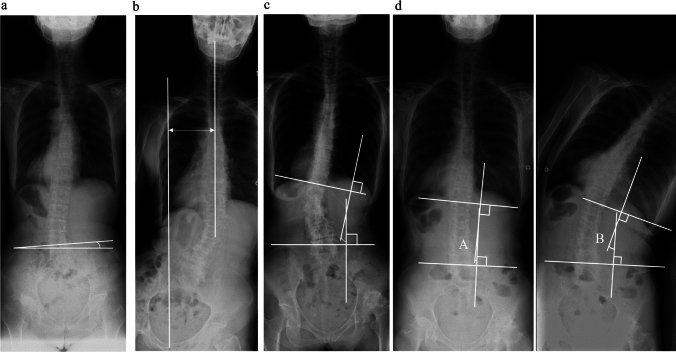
Spinal radiographic parameters. a Pelvic obliquity angle (POA). b Cervical 7 coronal vertical axis (C7CVA). c Lumbar scoliosis angle. d Lumber bending range (LBR)
